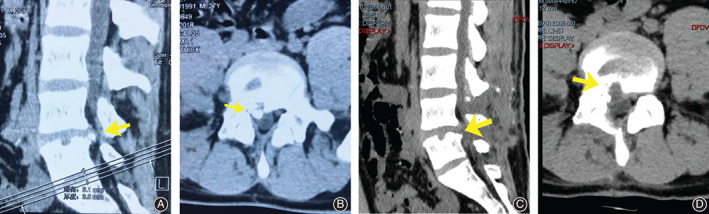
A 27‐year‐old male patient was hospitalized for low back pain and right lower extremity pain for 4 months. (A & B) Preoperative CT images; his diagnosis was L_4–5_ posterior ring apophyseal fracture. (C & D) Postoperative CT images. After FD, the bone was removed, and the compression was released.
